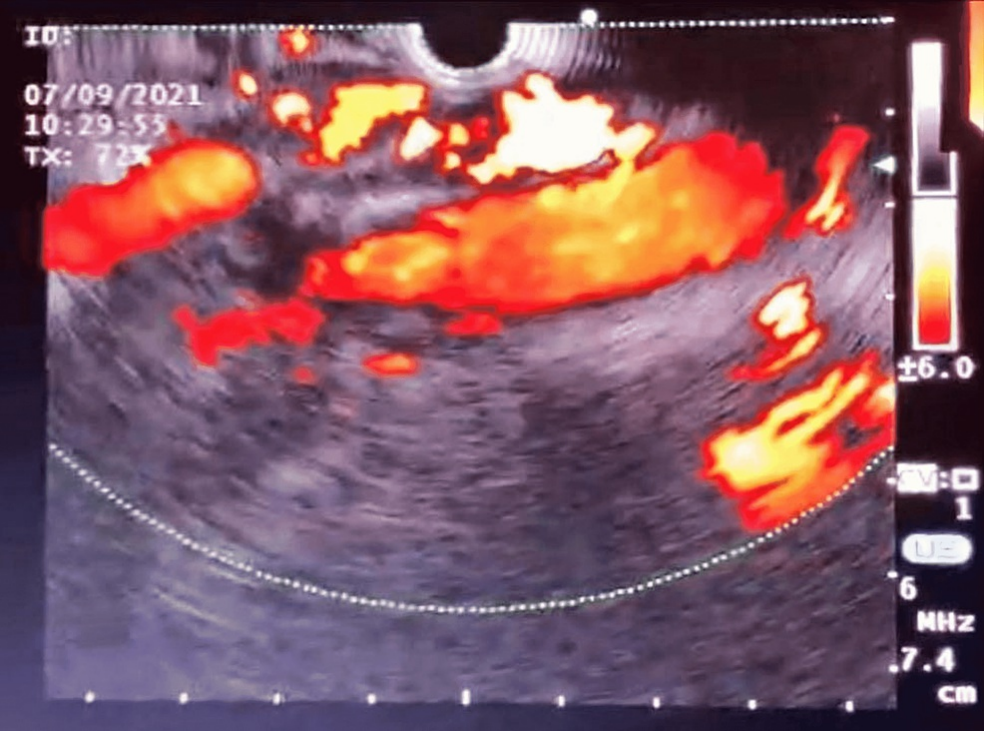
EUS image showing intense doppler signals of the vascular lesion around the celiac artery take-off
